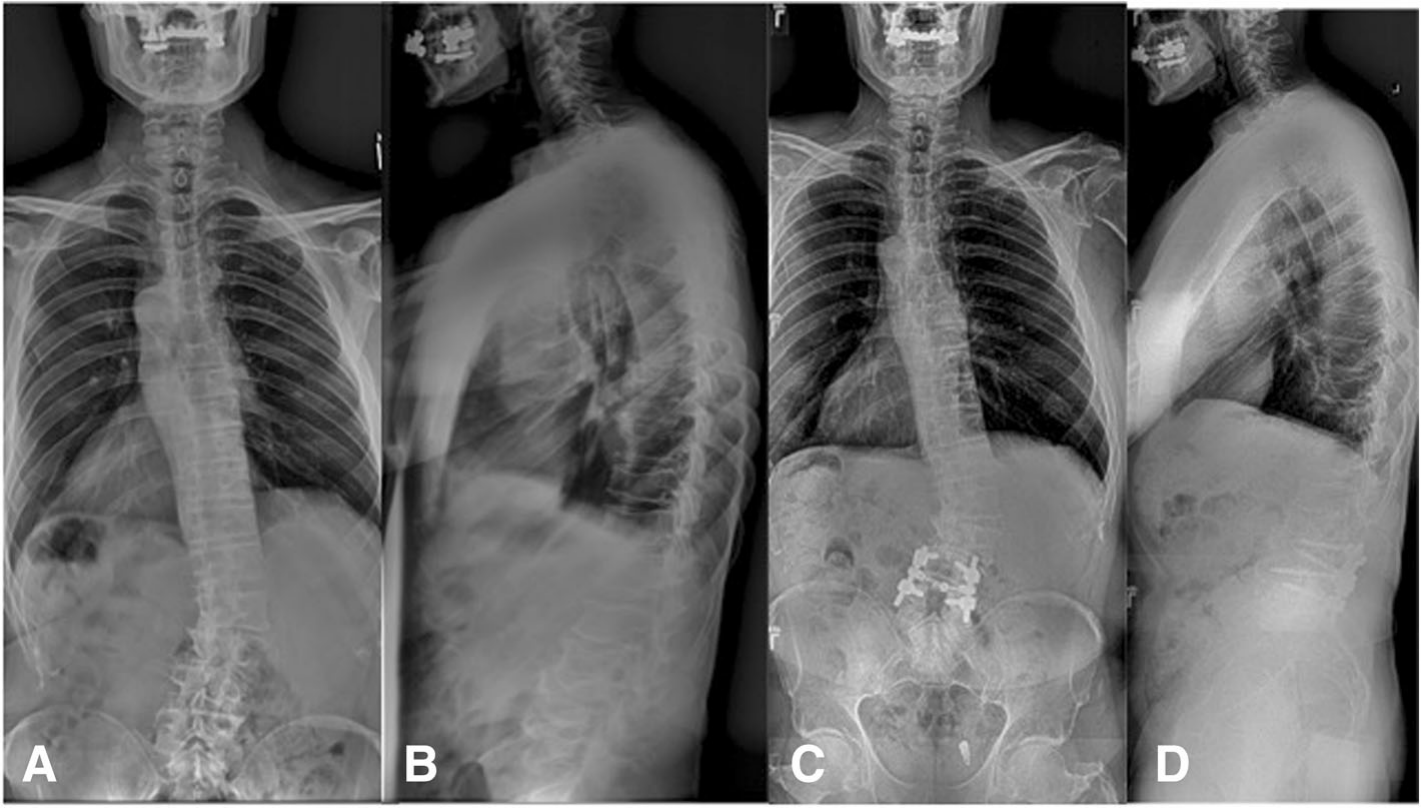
Anteroposterior (AP) (a) and lateral (b) full-length spine radiographs of a 63-year-old male that presented with mild axial back pain and progressively worsening L4 radicular symptoms down the right lower extremity. After failing conservative measures, the goal of the surgery was to simply address the symptomatic levels. There was no significant deformity or instability. Post-operative AP (c) and lateral (d) full-length spine radiographs showing the laminectomy, foraminotomy, and posterolateral fusion that was performed at L4-5
